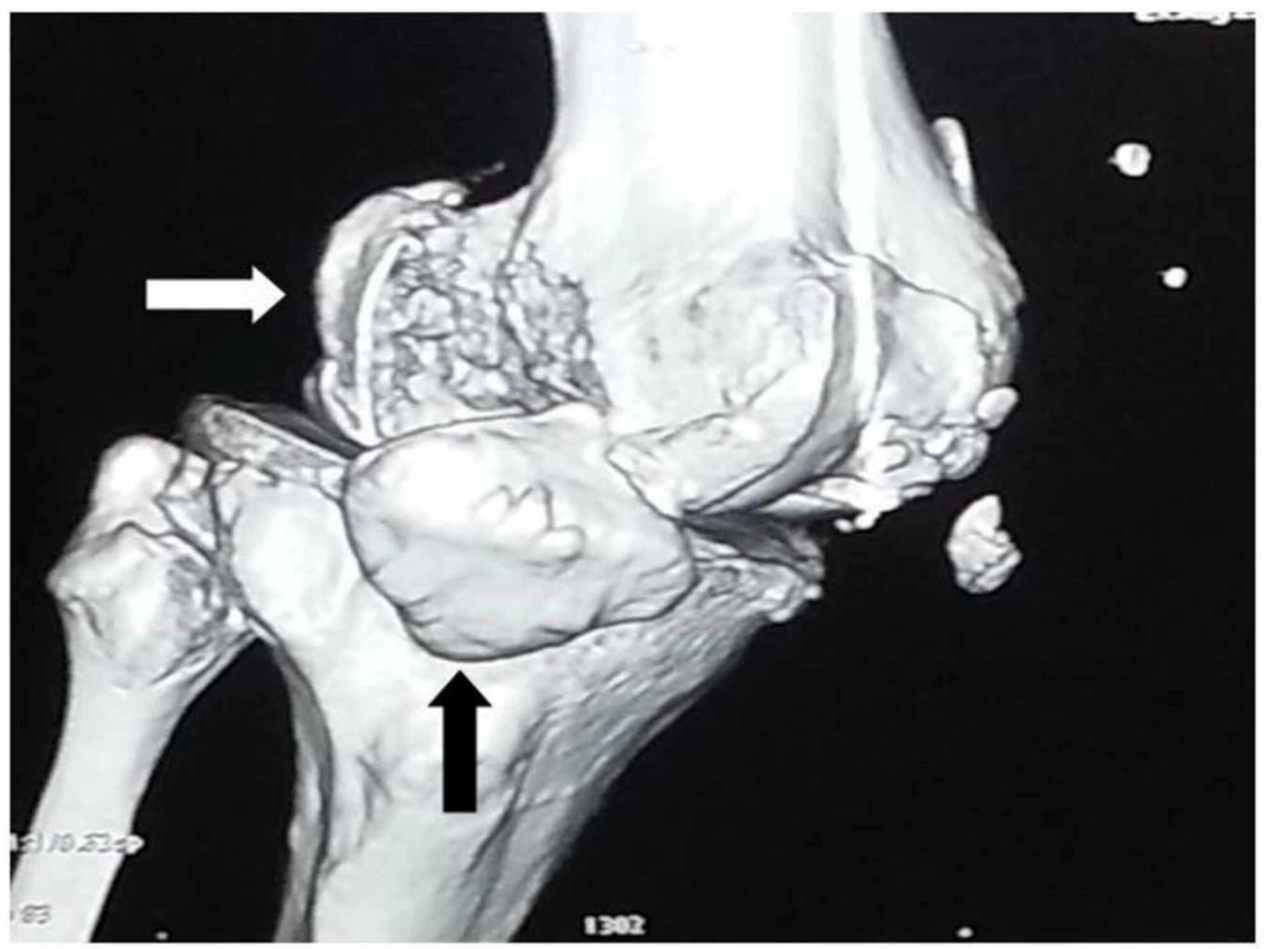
3-D computed tomography showing Hoffa’s fracture (white arrow) with patella dislocation (black arrow)
